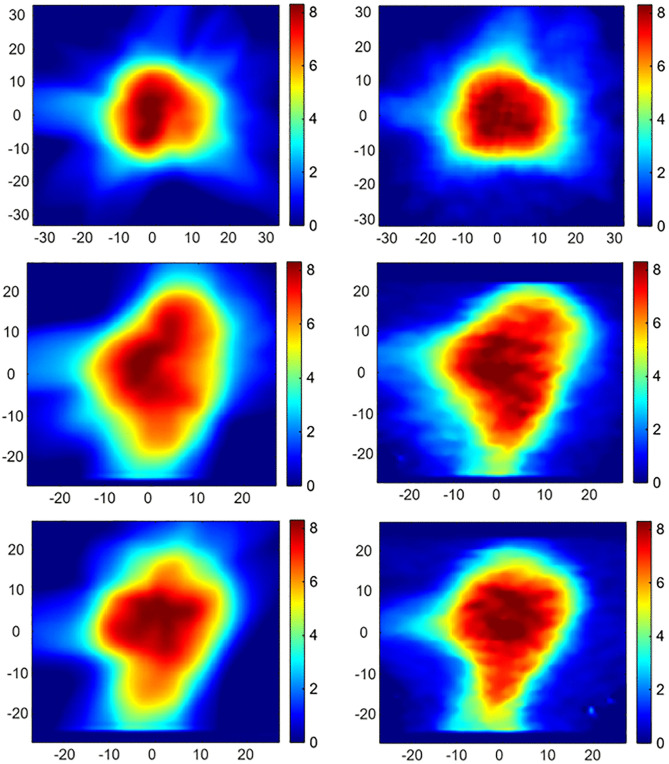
Spatial dose distributions obtained from the treatment planning system (TPS) and the MRI-derived R2 maps of the ACAGLPVA gel dosimeter. The left and right columns show the TPS-calculated and R2-MRI–derived dose distributions, respectively. The top, middle and bottom rows correspond to the central axial, coronal and sagittal slices, respectively. The color scale represents dose values ranging from 0 to 8 Gy.
